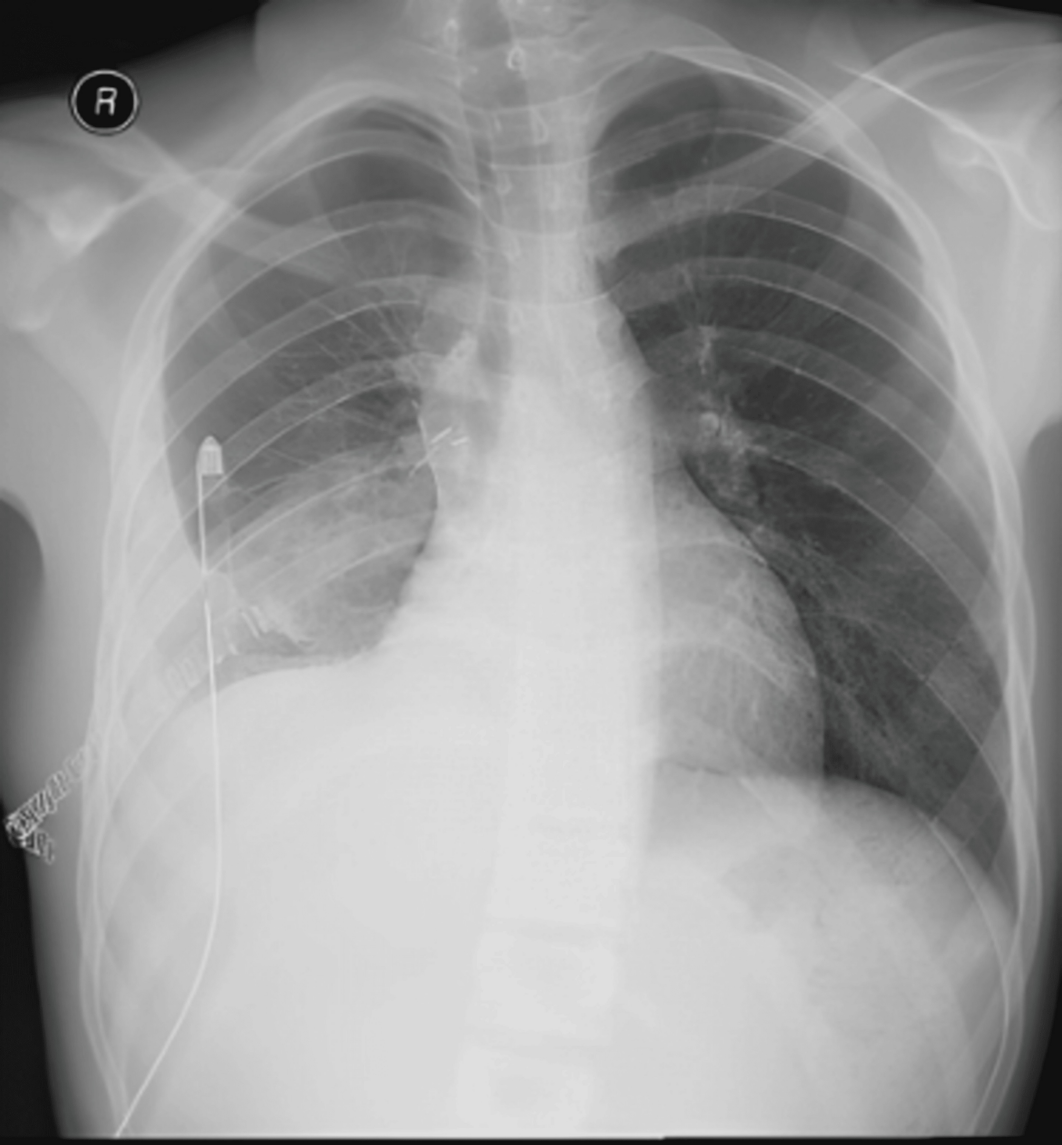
Postoperative chest X-ray with right-sided chest tube
